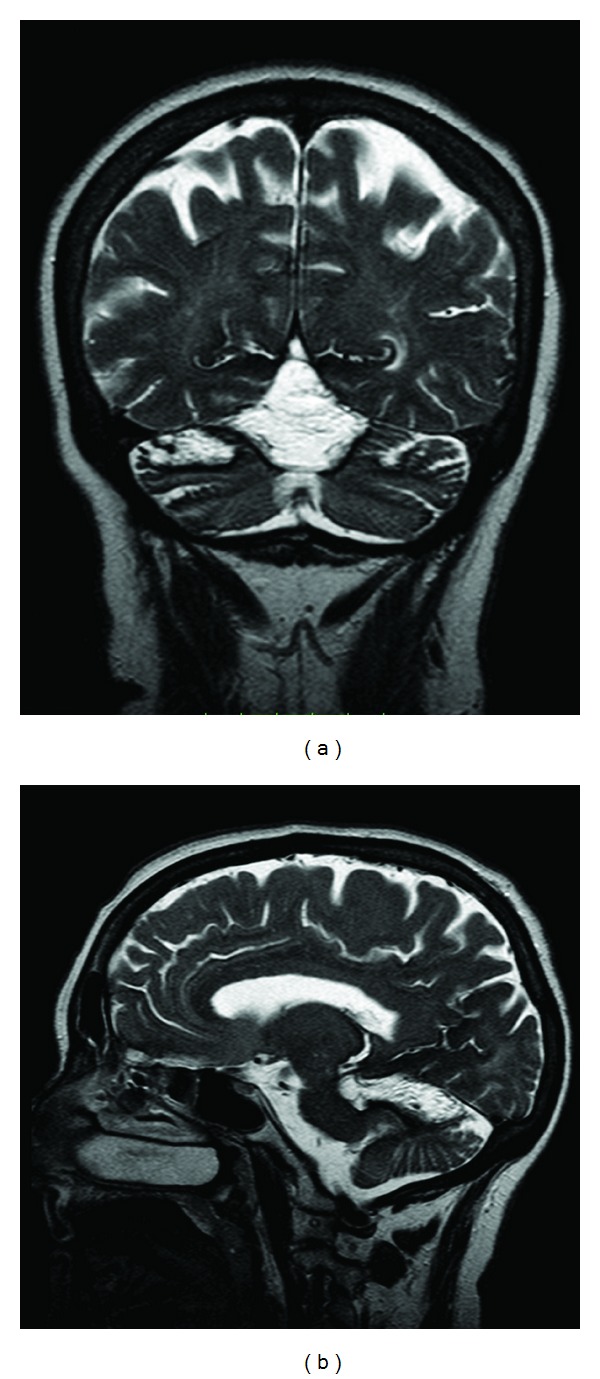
The coronal (a) and parasagittal (b) T2-ponderation MRI from patient 1 show a large clastic lesion over the vermis, marked cerebellar atrophy, and iron-related hypointensities around cerebellar hemispheres and brainstem.
